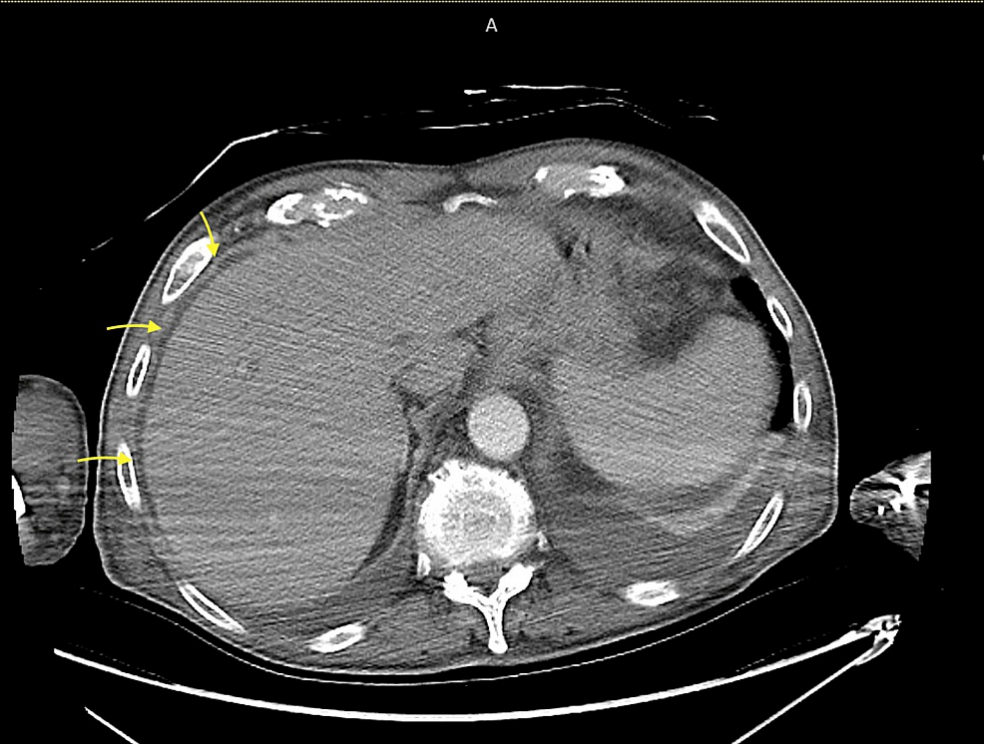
CT of the abdomen: axial view A small amount of peri-hepatic fluid is seen (indicated with yellow arrows) CT: computed tomography
